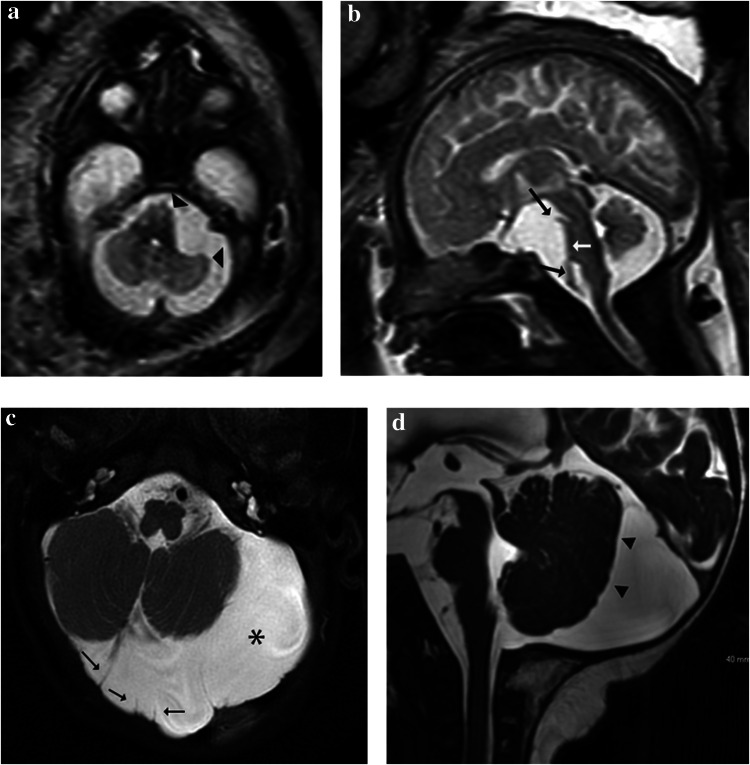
Arachnoid cysts. Left cerebellopontine angle arachnoid cyst diagnosed by fetal MRI (a) at 27 4/7 weeks on axial T2 SSFSE based on mass effect upon the left pons and anterior cerebellar hemisphere (*arrowheads*). Prepontine arachnoid cyst diagnosed by fetal MRI (b) at 33 4/7 weeks on sagittal T2 SSFSE based on mass effect upon the ventral pons (*white arrow*) and dorsal displacement of the basilar artery flow void (*black arrows* in b). Based on its location between the chiasmatic, interpeduncular, and prepontine cisterns, this represents a “membrane of Lilliquist” arachnoid cyst. c, d Postnatal brain T2-weighted MRI of a different patient at age 6 months with posterior fossa arachnoid cyst. Axial (c) and sagittal (d) images demonstrate mass effect upon the left cerebellum and upon the vermis posteriorly (*arrowheads* in d) with unilateral expansion of the left posterior fossa fluid space (*asterisk*). Normal falx cerebelli is present in the inferior midline cisterna magna (*arrows* in c)
